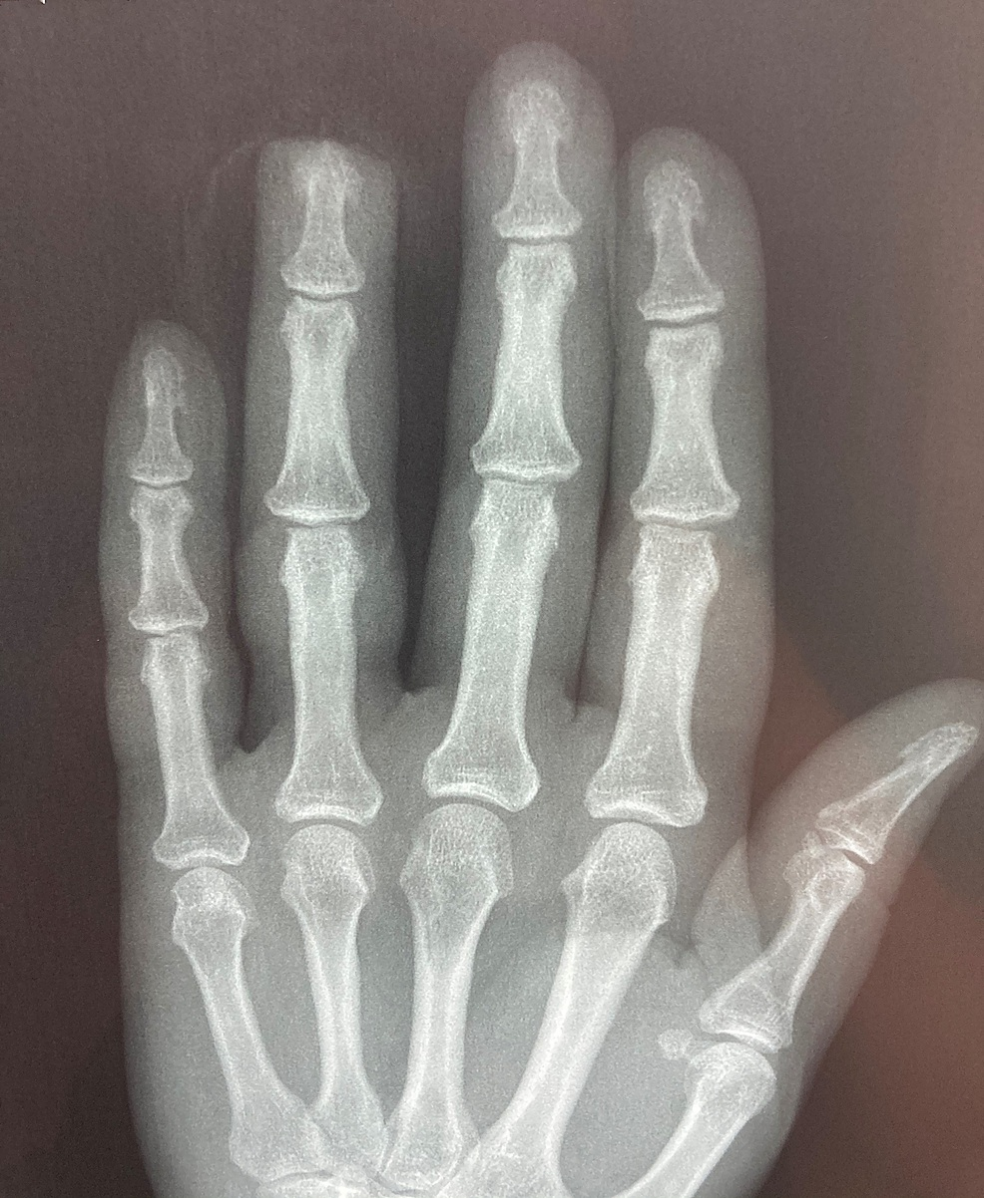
Anteroposterior radiographs of the hand showing the soft tissue injury of the ring finger.
